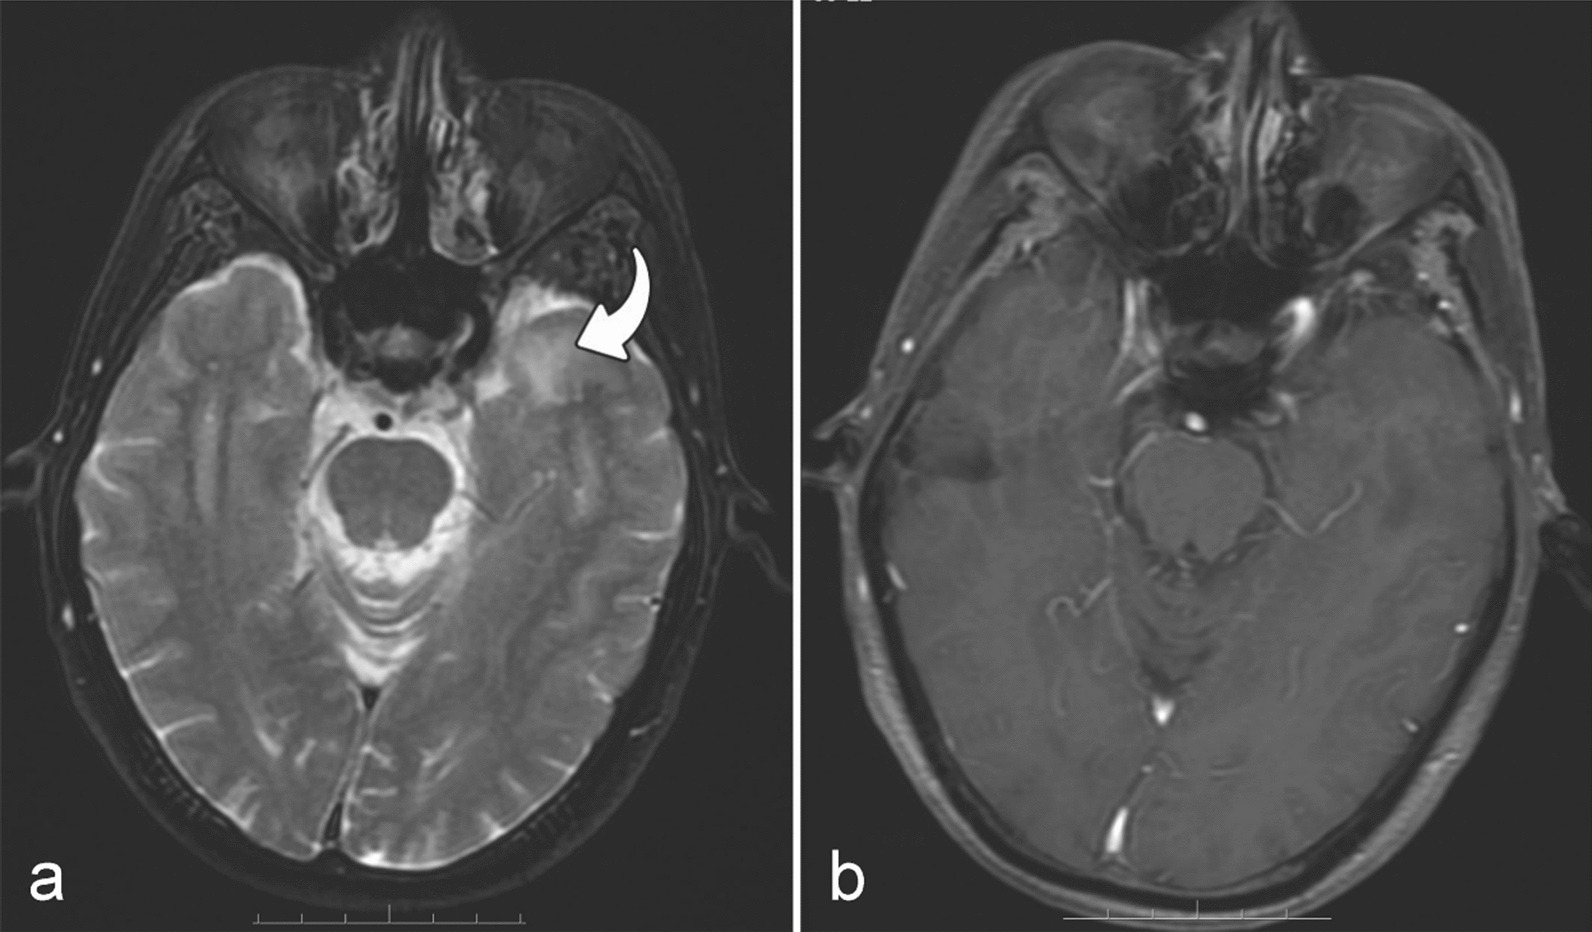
MRIs of a patient with RTLI (65y, F). MRIs of a patient with RTLI (65y, F) with the earliest MRI findings of isolated white matter lesion at 15.33 months after receiving IMRT. Axial T2-weighted image shows high intensity in white matter (curved arrow) of the left temporal lobe (a), but no significant enhancement is observed on the axial post-contrast T1-weighted image (b). *Note*: *MRI* magnetic resonance imaging, *RTLI* radiotherapy-induced temporal lobe injury, *IMRT* intensity-modulated radiotherapy
